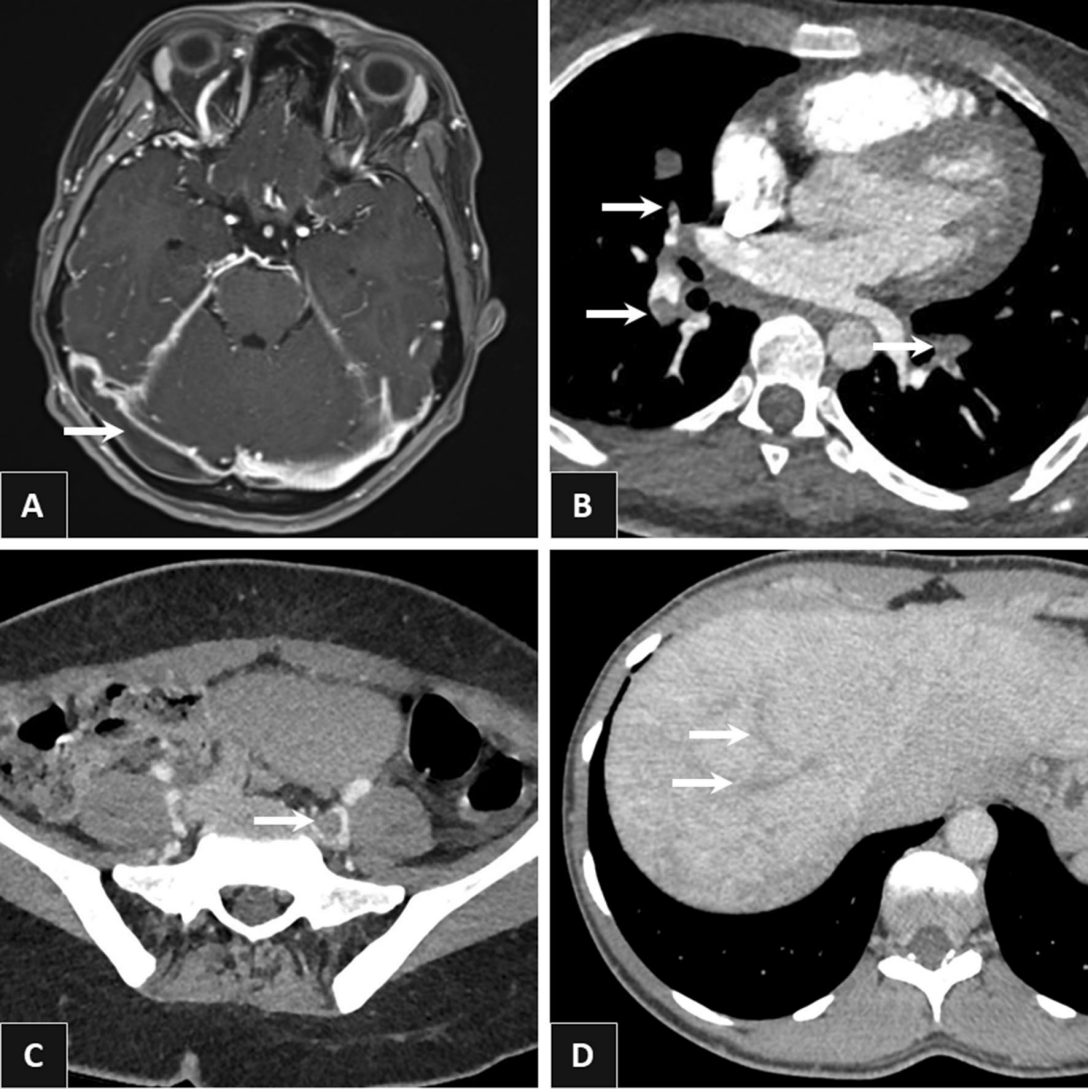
Various examples of eosinophil-related venous thrombosis. Right transverse sinus thrombosis on brain Magnetic Resonance Imaging (A), bilateral pulmonary embolism (middle lobe medial segment, right posterior basal segment left anterior basal segment) on Computed Tomography pulmonary angiography (B), left common iliac vein thrombosis on Computed Tomography venography (C), right and middle hepatic thromboses on contrast-enhanced abdominal Computed Tomography at late portal phase (D).
